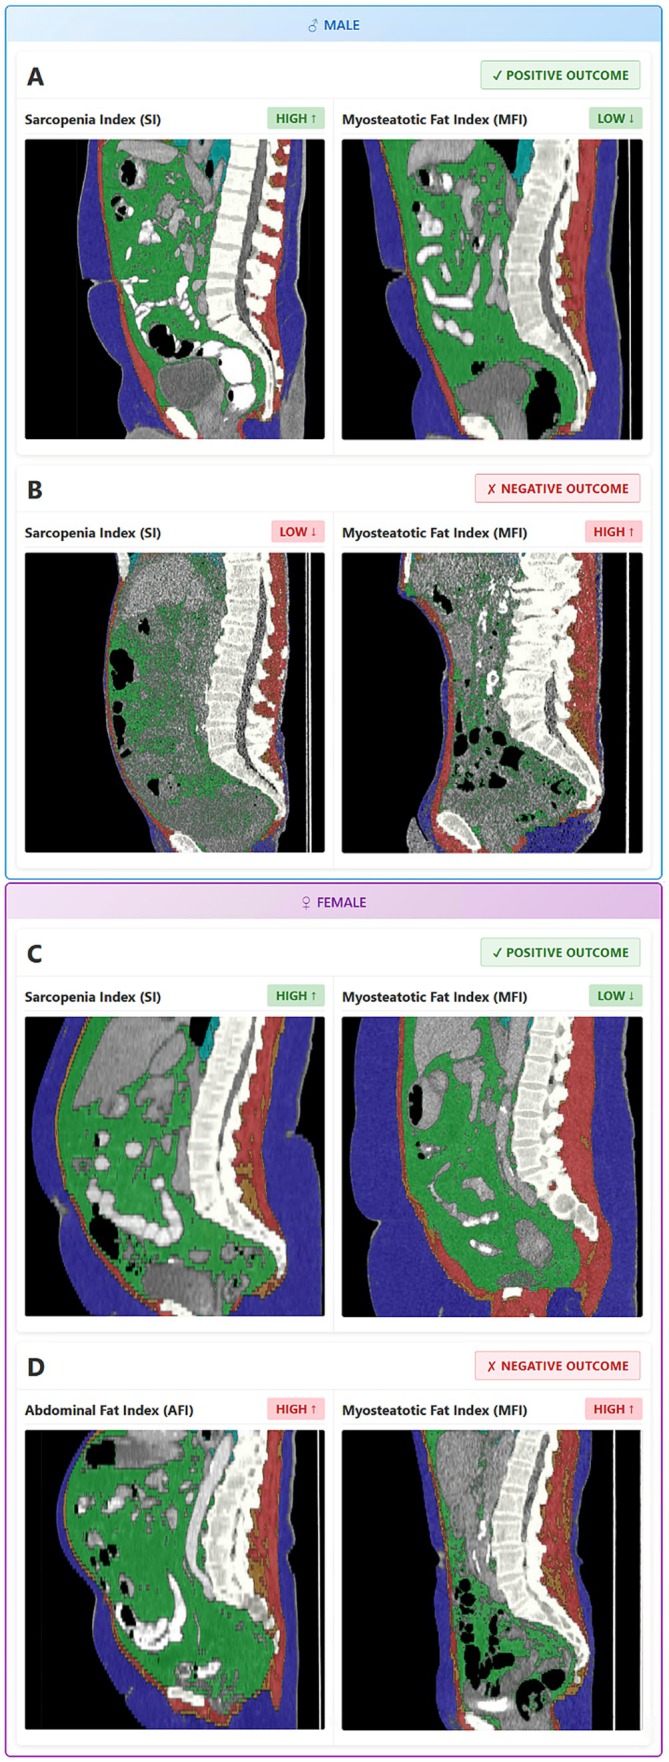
Representative sagittal CT images from the study cohort demonstrating imaging parameters stratified by sex and clinical outcome. Each parameter is measured from a different representative patient. (A) Male patients with positive outcome demonstrating high sarcopenia index (SI) and low myosteatotic fat index (MFI) (SI: 46y AC, T3N1M0, G2, OS 59.3 months, full remission; MFI: 69y adenocarcinoma, T3N2M0, G3, OS 22.8 months, deceased). (B) Male patients with negative outcome demonstrating low sarcopenia index (SI) and high MFI (SI: 58y epithelial carcinoma, T1N0M0, unspecified, OS 1.2 months, deceased; MFI: 67y squamous cell carcinoma, T3N1M0, G2, OS 6.6 months, deceased). (C) Female patients with positive outcome demonstrating high Sarcopenia Index (SI) and low MFI (SI: 56y adenocarcinoma, T2N0M0, G3, OS 172 months, full remission; MFI: 51y adenocarcinoma, T3N1M0, G3, OS 51.3 months, full remission). (D) Female patients with negative outcome demonstrating high abdominal fat index (AFI) and high MFI (AFI: 82y adenocarcinoma, T3N1M0, G3, OS 9.5 months, deceased; MFI: 60y squamous cell carcinoma, T3N0M0, G2, OS 49.2 months, deceased).
